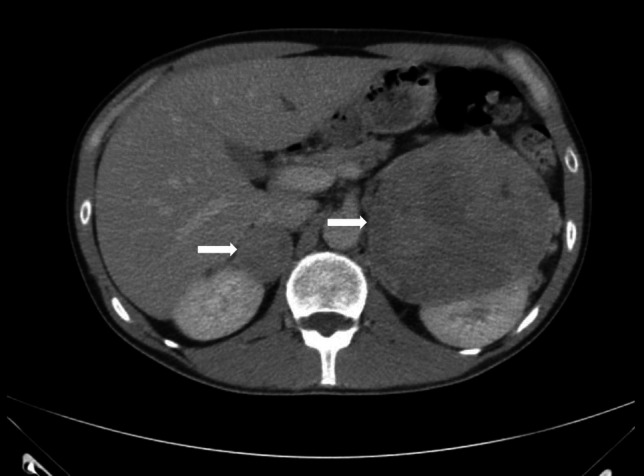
CT scan showing bilateral adrenal masses
